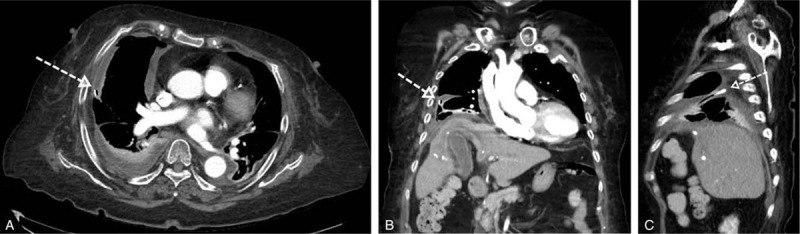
(A): Axial scan (B): Coronal scan (C): Sagittal scan. Right pleural empyema markedly decreased on axial, sagittal scan (A, C) of the follow-up CT. Also, the liver abscess markedly decreased, and curvilinear low attenuated lesion in the right lobe of the liver was still visible on the coronal CT scan (B). There is a chest tube in the right pleural space (empty arrow).
